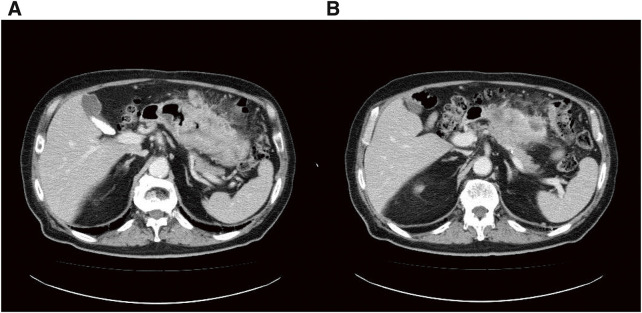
Contrast-enhanced CT findings. The contrast-enhanced CT showed wall thickening in the body of the stomach, as well as (A) peritoneal disseminated nodules and (B) pancreatic infiltration. CT, computed tomography
